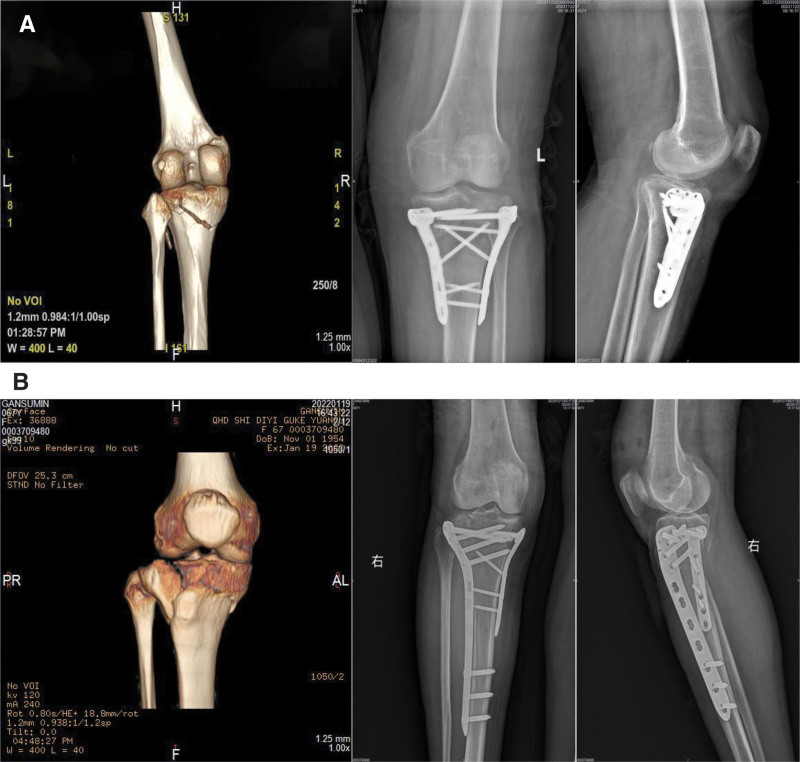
Figure (A) shows the preoperative and postoperative X-ray images of a patient with a tibial plateau fracture treated with compression fixation, and (B) shows the preoperative and postoperative X-ray images of another patient with a tibial plateau fracture treated with compression fixation.
